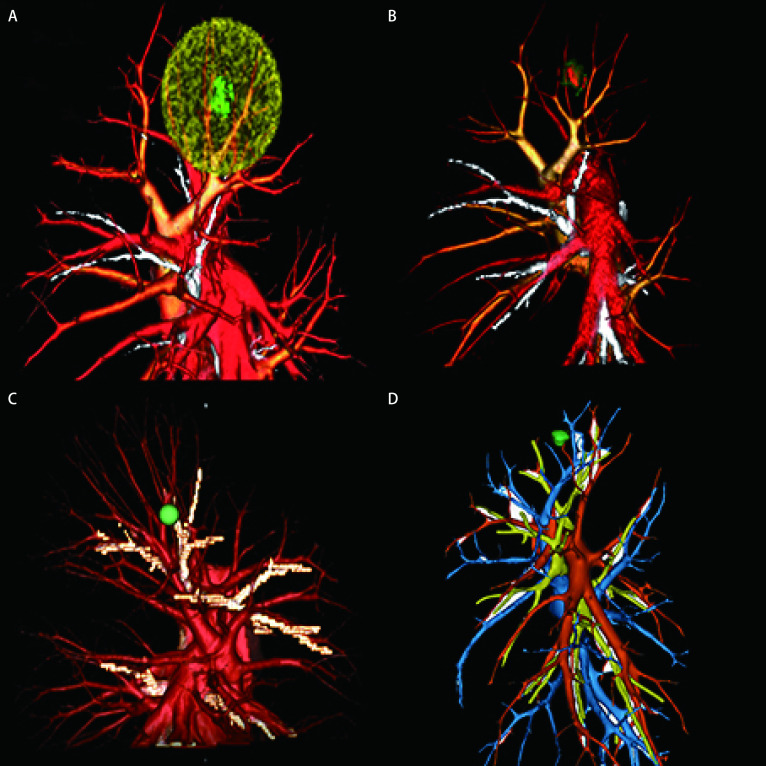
三维重建技术。A：切缘球制定手术规划；B：部分实性结节三维特征，红色代表实性成分，绿色代表磨玻璃成分；C：Horos对CT成像质量要求低，无法直接区分动静脉；D：Mimics操作时间长，对解剖知识储备要求高，动静脉区分度好。 3D reconstruction technology. A: Cutting edge balls for surgical planning; B: Part solid nodules three-dimensional reconstruction, red part represents solid components and green represents ground glass components; C: Horos has low requirements on CT imaging quality and cannot distinguish arteriovenous directly; D: Mimics needs long operation time, high requirement for anatomical knowledge reserve and good differentiation of arteriovenous. CT: computed tomography
